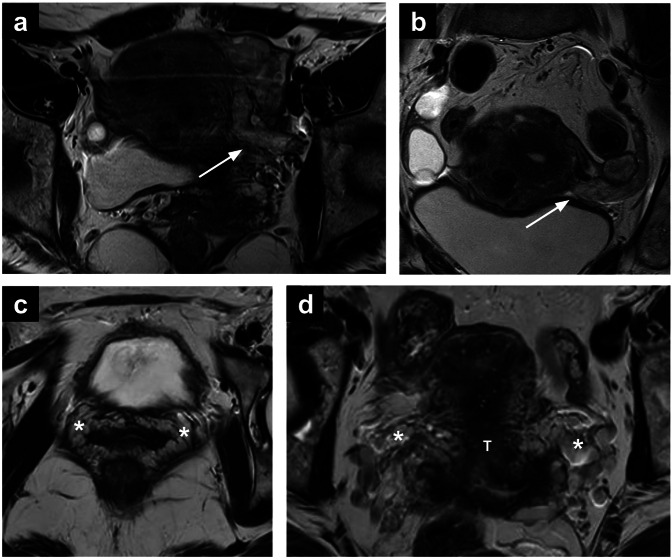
Intravascular leiomyomatosis (a, b). Axial and coronal T2-WI (a, b) showing incidental finding of left intravascular leiomyomatosis (arrow) in a 44-year-old patient who underwent MRI for characterisation of multiple uterine leiomyomas. The lesion has an elongated shape and intermediate-to-high SI on T2-WI. Pelvic congestion (c, d). Axial and oblique coronal T2-WI (c, d) showing bilateral pelvic congestion in a 46-year-old patient undergoing MRI for cervical cancer (T) staging. If parametrial invasion is absent and vessel enlargement is symmetric (like in this case), pelvic congestion is the most likely diagnosis. SI, signal intensity; WI, weighted images
